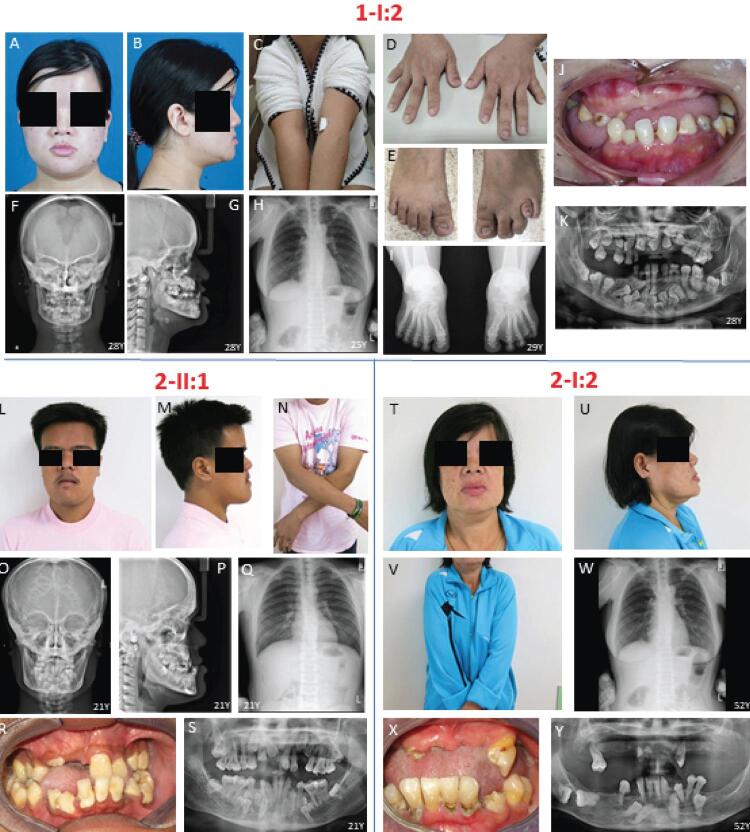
Clinical and radiographic features of 1-I:2, 2-II:1, and 2-I:2. (A-K) 1-I:2 shows typical CCD craniofacial features, narrow shoulder, and brachydactyly and short fourth toe of the left foot. Radiographs shows hypoplasia of the lateral part of both clavicles, widened cranial sutures, Wormian bones, fourth-ray brachymetatarsia of the left foot, unerupted teeth, and supernumerary teeth. (L-S) 2-II:1 shows normal clavicles, CCD facial features with asymmetry, widened cranial sutures, Wormian bones, spina bifida in the lower cervical spine, scoliosis of the lower thoracic spine, supernumerary teeth. (T-Y) 2-I:2 has unerupted teeth and normal clavicles
